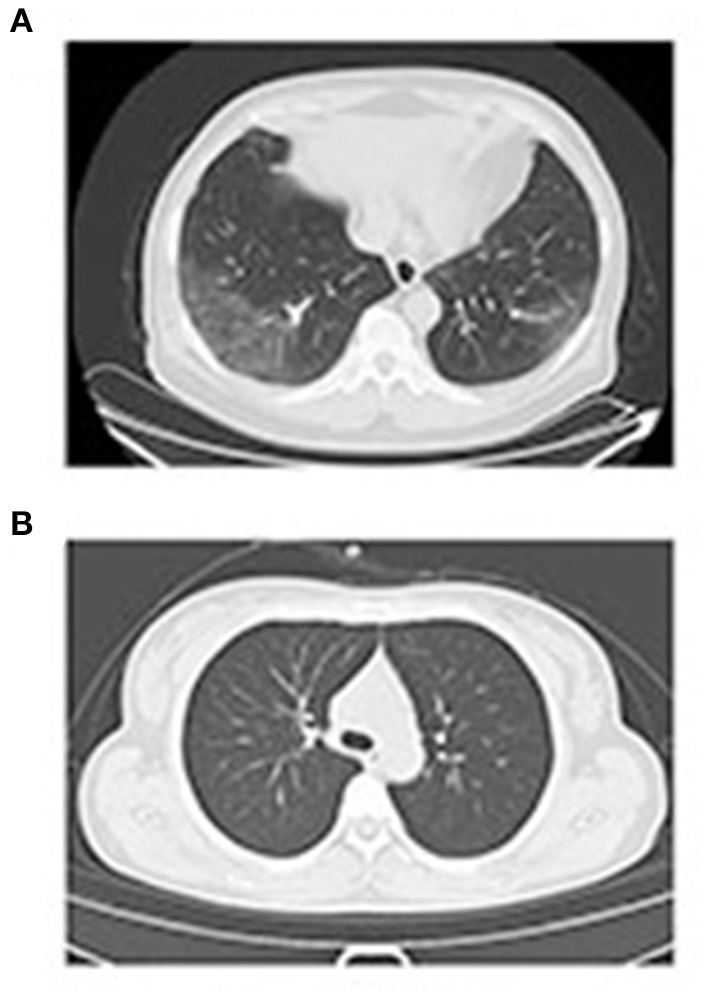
Patient case CT scan. (A) For patient 55, the nucleic acid detection result was negative, but the IgM and IgG results were positive. In the lower regions of both lungs, large fuzzy shadows, GGO, and a slightly fan-shaped distribution were observed. (B) In patient 19, the nucleic acid detection result was positive, but the IgM and IgG results were negative, and no clear lesions were found in the lungs.
